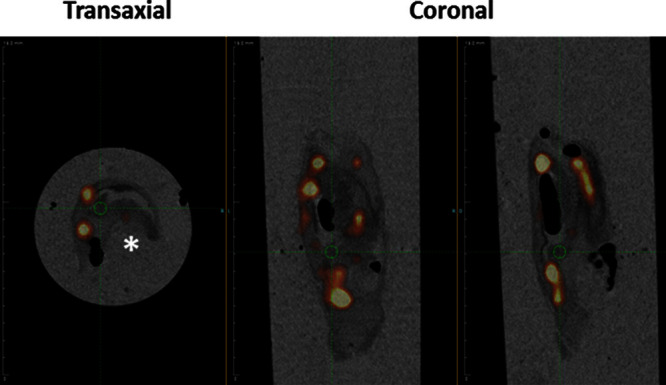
Ex vivo combined positron emission tomography/computed tomographic visualization of a plaque recovered from the internal carotid artery demonstrating heterogeneous [^64^Cu] [1,4,7,10-tetraazacyclododecane-*N*,*N*′,*N*″,*N*‴-tetraacetic acid]-*d*-Phe1,Tyr3-octreotate (^64^Cu-DOTATATE) uptake 23 hours post injection. Left, Transaxial projection of plaque exhibiting hot spots of ^64^Cu-DOTATATE accumulation. Middle and right, Coronal projections at 2 different levels. * indicates residual vessel lumen.
